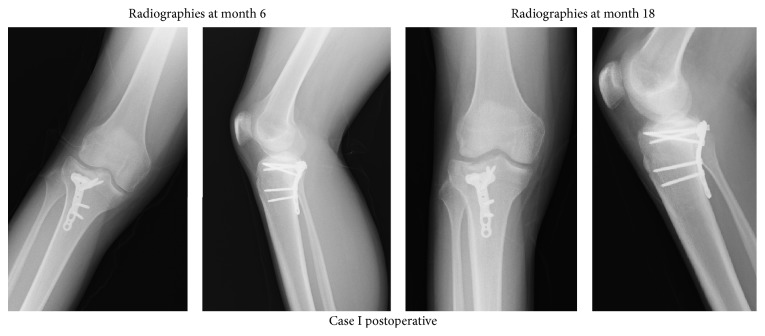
Postoperative radiographies of Case I.
